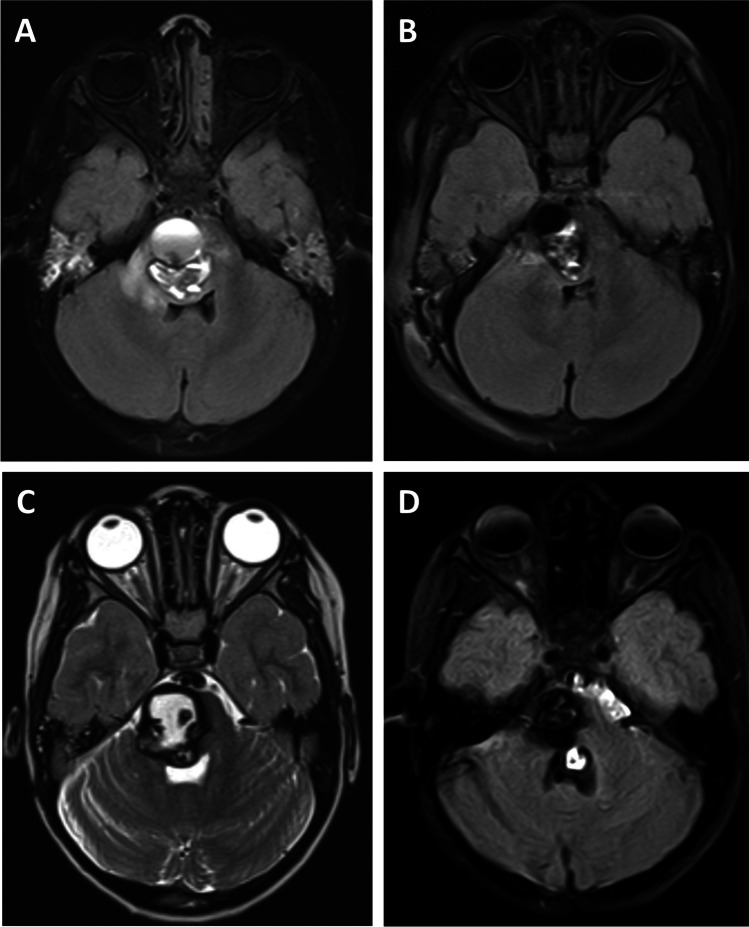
3-year-old female patient with symptomatic hemorrhage of a large CM located in the pons (A). After hematoma evacuation and partial resection of the CM via a retrosigmoidal approach (B), the patient recovered and developed well. One year later, asymptomatic progression was seen during a routine follow-up MRI (C). Although the lesion was still evident during a follow-up MRI almost 4 years later (D), the patient experienced no further neurological symptoms since the last surgical intervention
